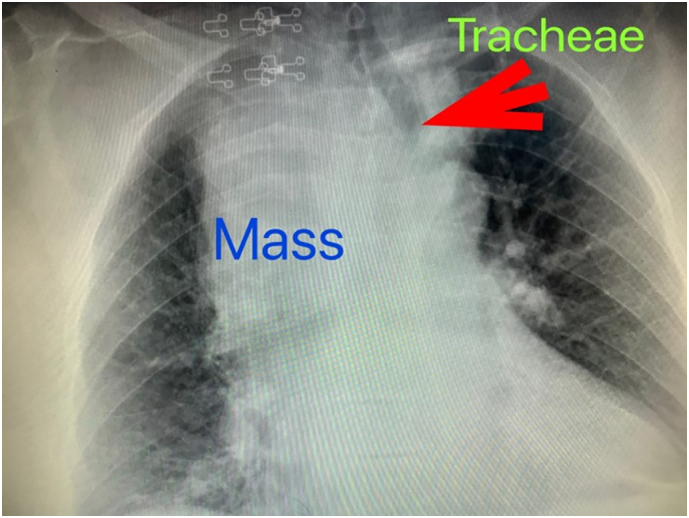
Chest x-ray demonstrating a widened mediastinum with tracheal deviation to the left.
